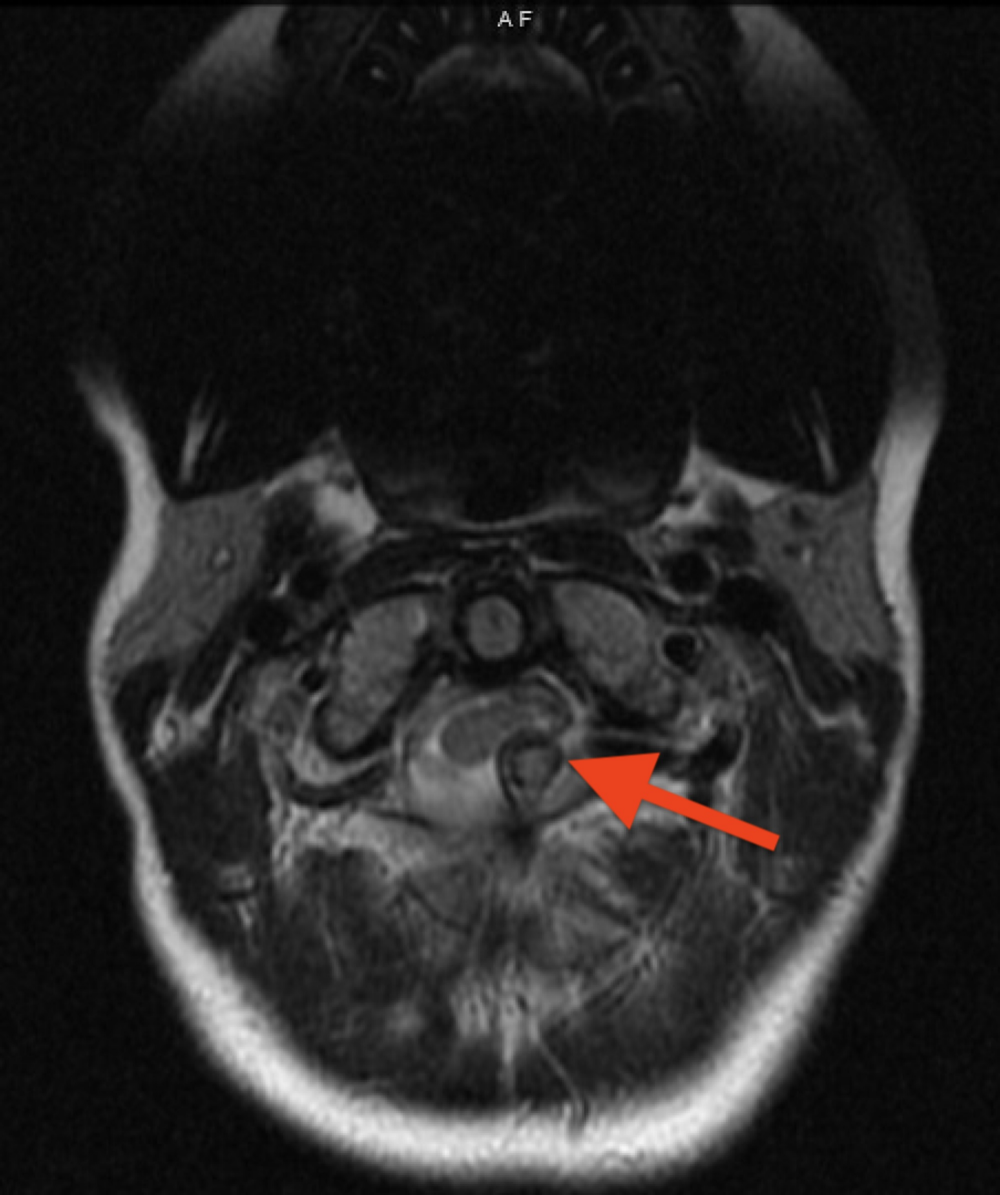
Cervical spine MRI axial view demonstrating an osteochondroma extending from the posterior arch of C1 and compressing the spinal cord
